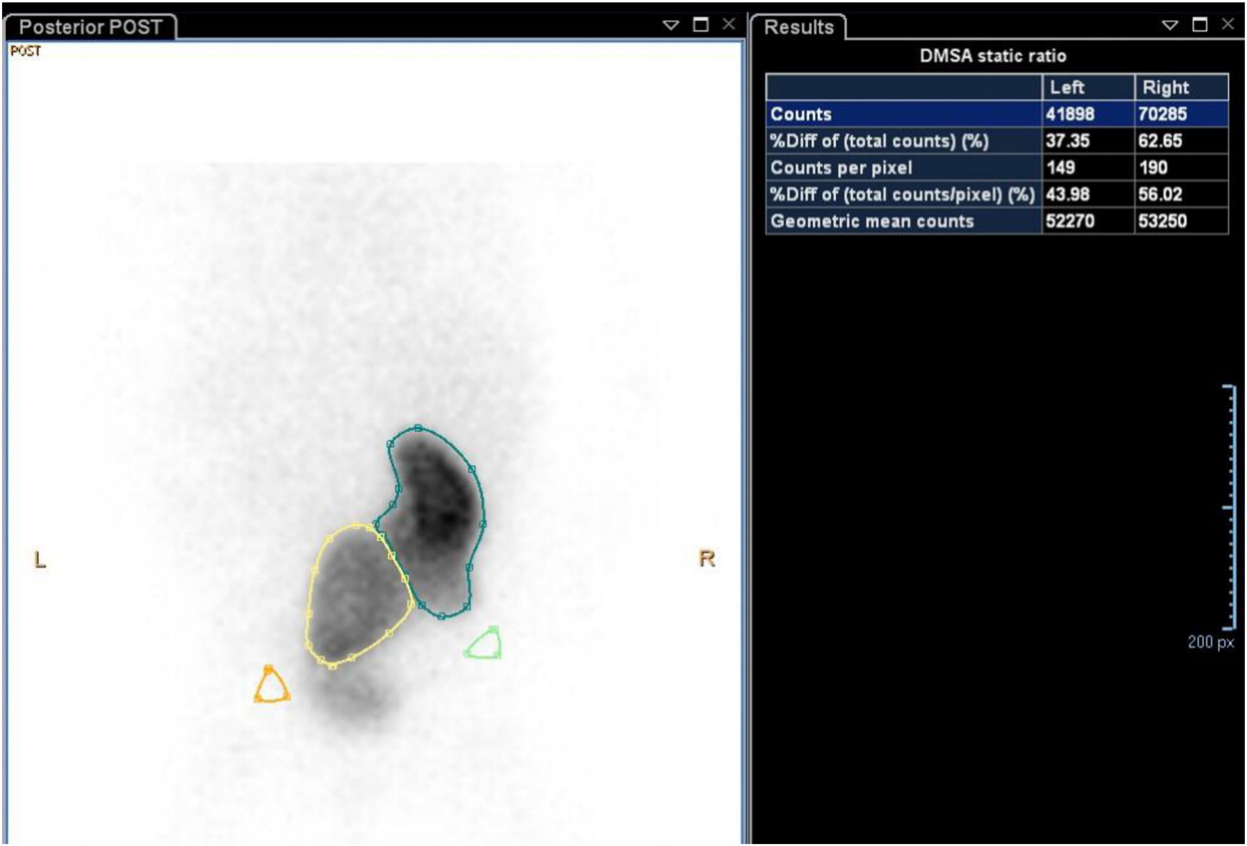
Dimercaptosuccinic acid (DMSA) renal cortical scintigraphy demonstrating a smaller, malrotated left pelvic kidney with homogeneous tracer uptake and a normally positioned right kidney with intense cortical uptake, without evidence of scarring (August 18, 2025).
